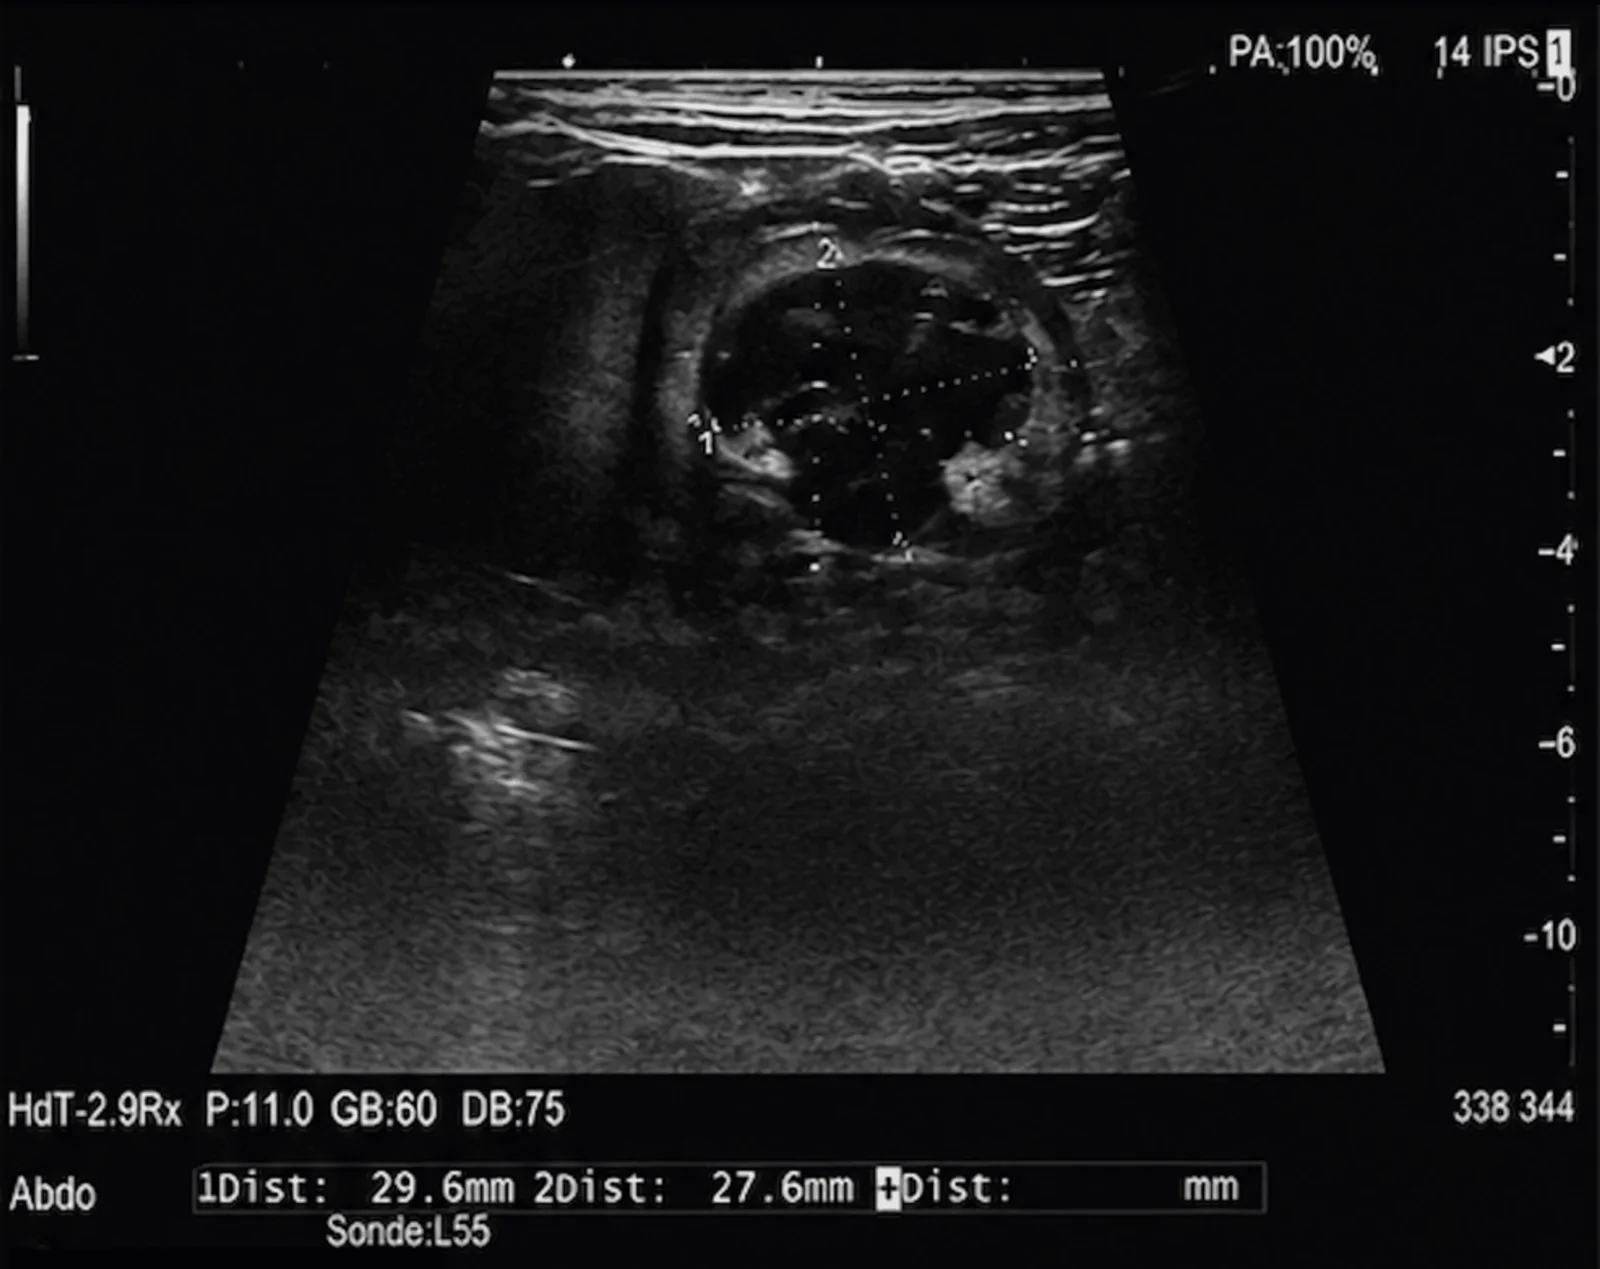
Follow-up abdominal ultrasound. Small necrotic-appearing residual retroperitoneal mass.
